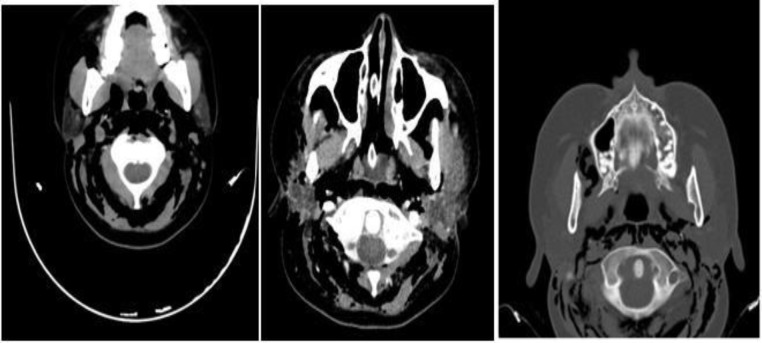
Computed tomography scan of paranasal sinuses showing emphysema in parietal, temporal, and occipital soft tissue and retropharyngeal space
